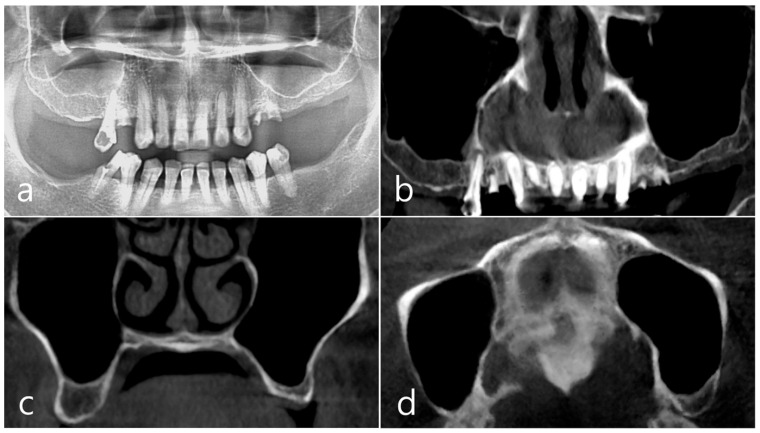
Case 1. (a) In panoramic radiography, resorption of residual alveolar ridge and pneumatization of the maxillary sinus were observed in the left and right maxillary posterior region; (b–d) In preoperative CBCT’s panoramic, coronal, and axial images, there were no abnormal findings of the maxillary sinus and no sinus membrane thickening.
